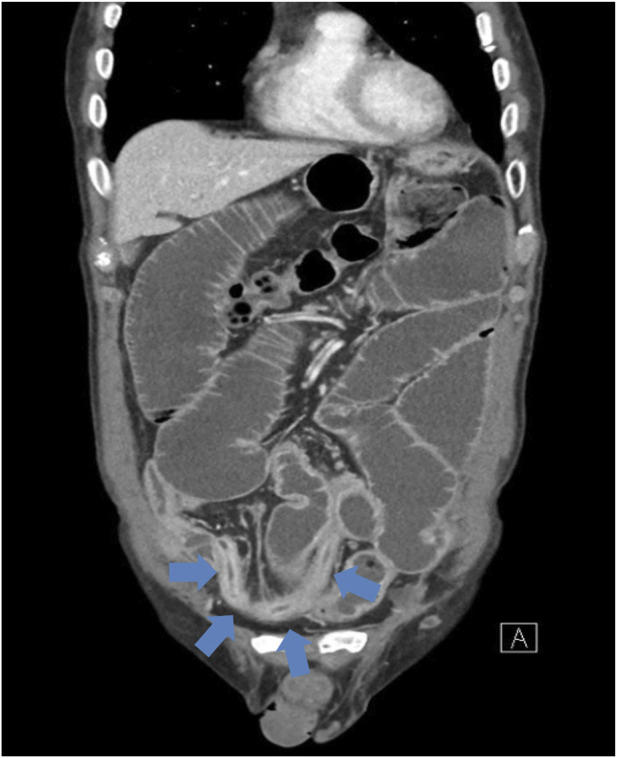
Coronal contrast-enhanced abdominal CT on HD 25 demonstrating multisegmental wall thickening and luminal narrowing from the distal jejunum to the distal ileum (arrows), compatible with inflammatory stricture and mechanical obstruction.
